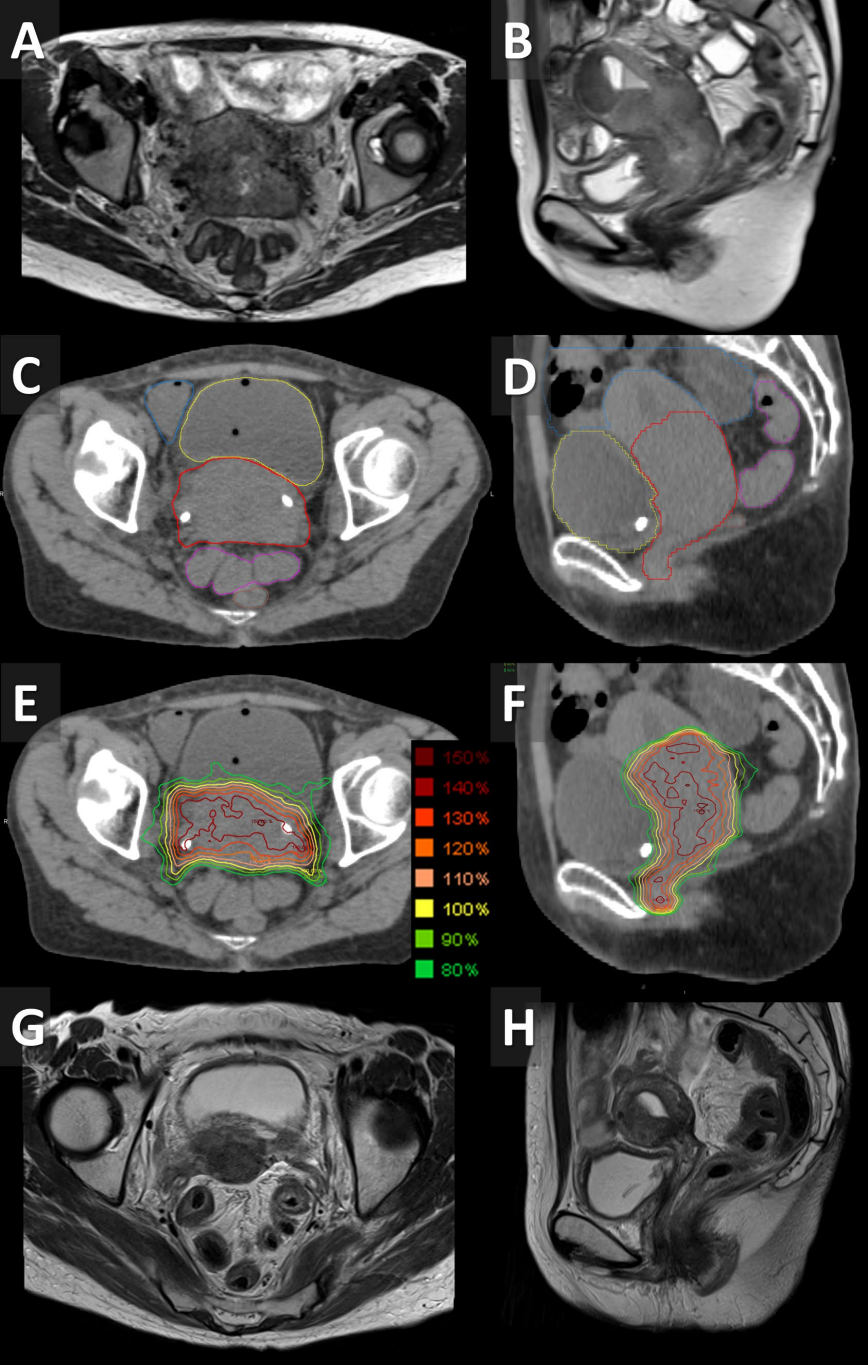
Images obtained from a 55-year-old woman with cervical cancer (T3bN1M0). A Axial and B sagittal T2-weighted MR images at SBRT initiation. C Axial and D sagittal CT images with contouring for planning SBRT (red = high-risk CTV). E Axial and F sagittal CT images with dose distribution of SBRT. G Axial and H sagittal T2-weighted MR images 3 months after SBRT. *CT* computed tomography, *CTV* clinical target volume, *MR* magnetic resonance, *SBRT* stereotactic body radiotherapy
